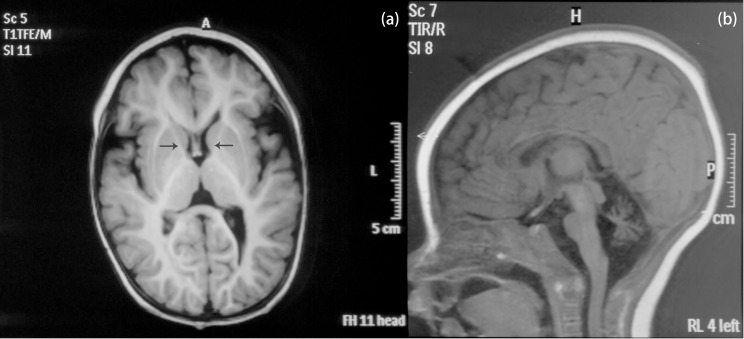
MRI result of the patient. (a) Coronal T1W image. Black arrows pointed the anterior limb of the internal capsule. The faint hyperintense signal of T1W indicated delayed myelination of the patient. (b) Sagittal T1W image. The patient presented cerebellar atrophy.
